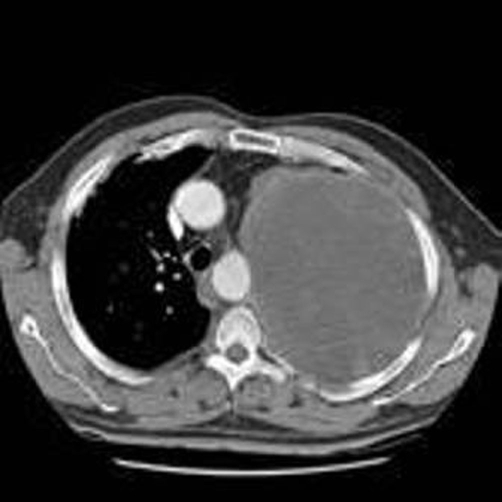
CT scan of carcinosarcoma of the left lung.
